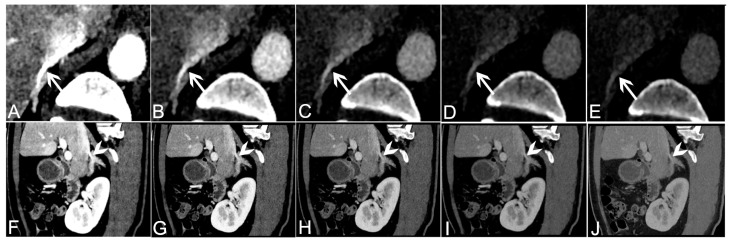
A 61-year-old male patient with a right adrenal adenoma underwent DE-CTA. Axial VMI+ reconstructions at a level of 40 keV (A), 50 keV (B), 60 keV (C), 70 keV (D) and 80 keV (E). The right adrenal vein (arrows) can be visualized. In this case, the right adrenal vein was filled with the contrast material at 40 keV. The CT value and CNR of the right adrenal vein at 40 keV were higher than those of the 50–80 keV VMI+ reconstructions. Sagittal images were reconstructed to visualize the right adrenal vein (arrowheads): 40 keV (F), 50 keV (G), 60 keV (H), 70 keV (I) and 80 keV (J). The clarity and observation of solid organs in 50 keV VMI+ reconstructions were better than those in other keV reconstructions. DE-CTA, dual-energy computed tomography angiography; VMI+, advanced virtual monoenergetic images; keV, kiloelectron volt; CNR, contrast-to-noise ratio.
